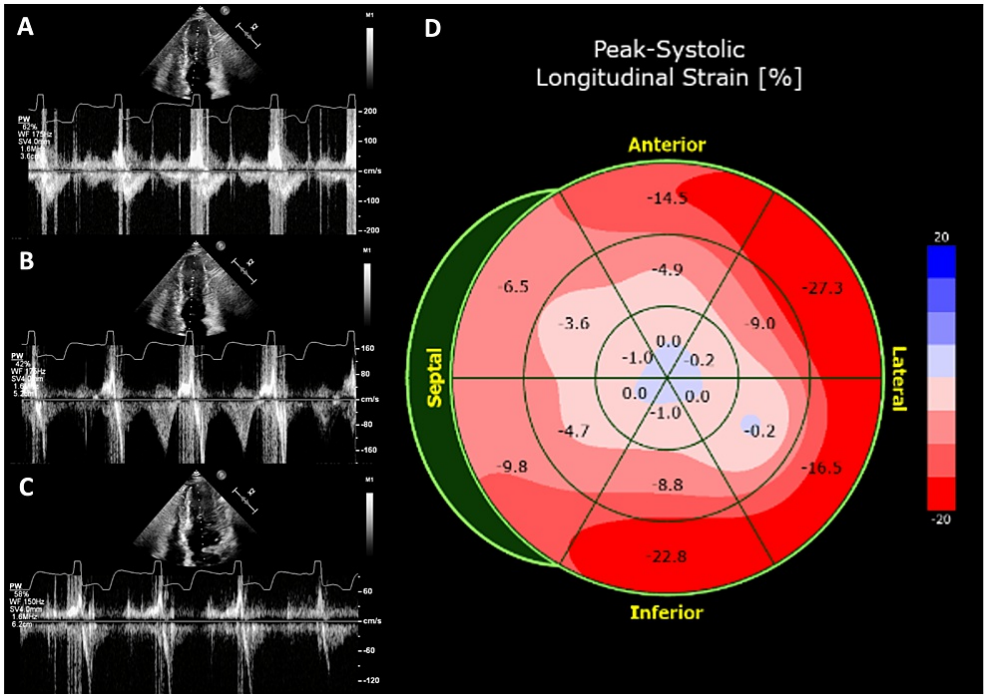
Echocardiographic evaluation 22 years after the OHT demonstrating the midventricular cavity obliteration (A-C) and strain polar plot (D) showing loss of longitudinal strain apically
